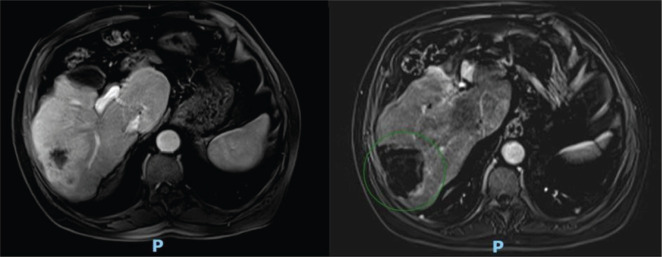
Clinical response of a right lobe liver lesion after microwave ablation. On the left, an axial pos-arterial contrast magnetic resonance imaging (MRI) of the upper abdomen with Primovist, from 05/16/2022, showing a hepatic mass in the posterior sector of the right lobe measuring 6.3 × 3.7 cm, with a large area of enhancement. On the right, a axial, post-arterial contrast subtraction MRI from 07/19/2022, with resolution of the enhancement area.
